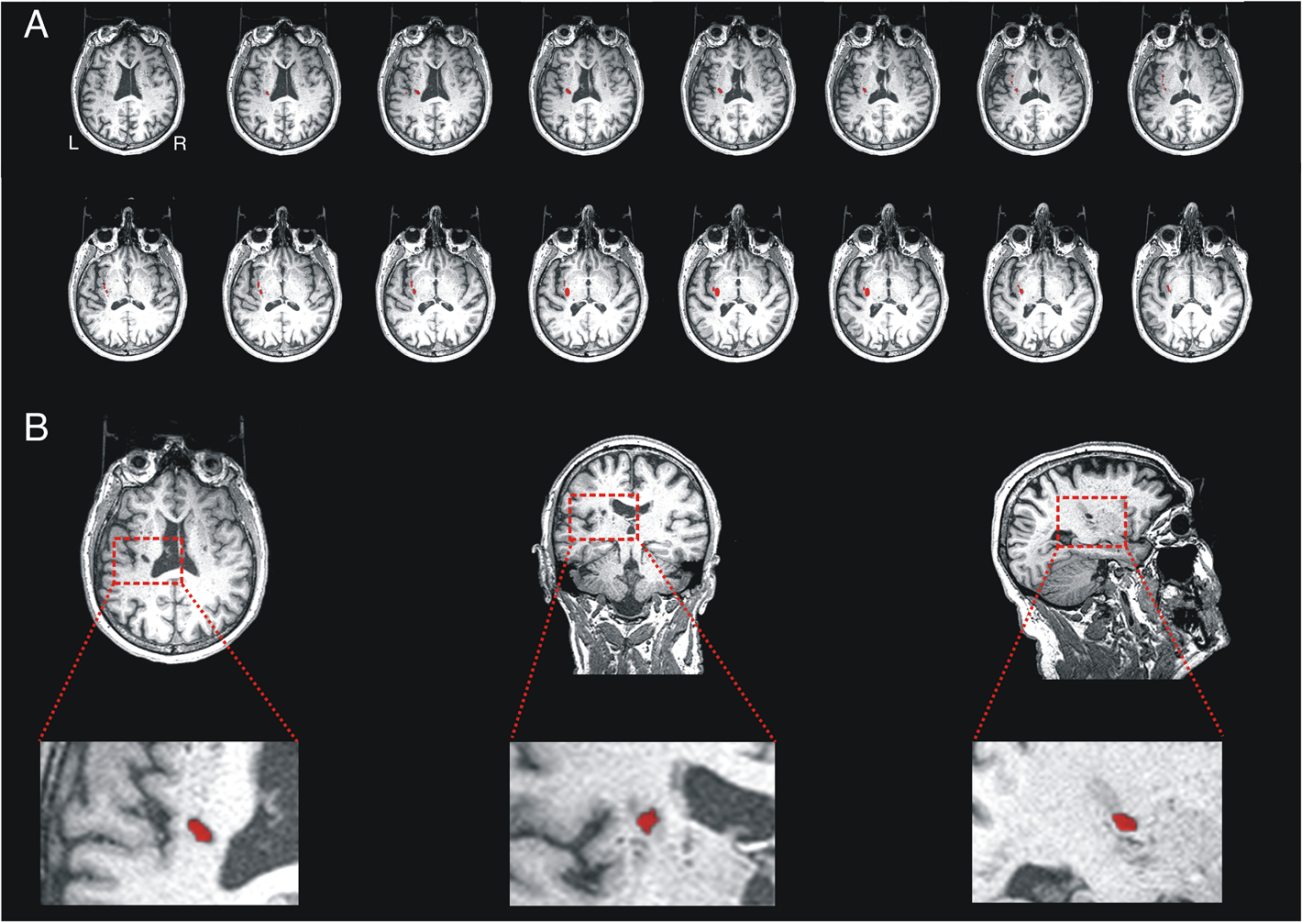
A. Slice-by-slice reconstruction of the axial T1-weighted image of the lesion. B. Axial, coronal and sagittal slices of the lesion, located on left hemisphere lesion at subinsular region level, including the left internal and external capsule, corona radiata and claustrum.
